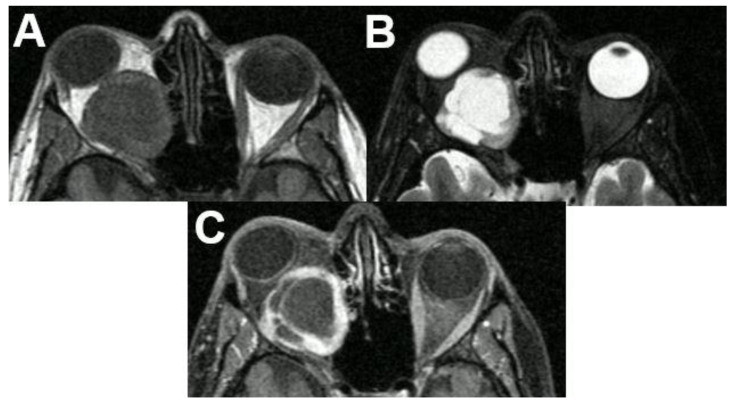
Schwannoma of the optic nerve.* MRI of a 36-year-old female presenting with a large retrobulbar tumor and exophthalmos. The tumor is hypointense on T1-weighted (A), hyperintense on T2-weighted sequences (B), and shows avid contrast enhancement of its solid parts (C). Note the cystic degeneration of the tumor. The tumor was surgically removed, and pathology revealed a schwannoma.
